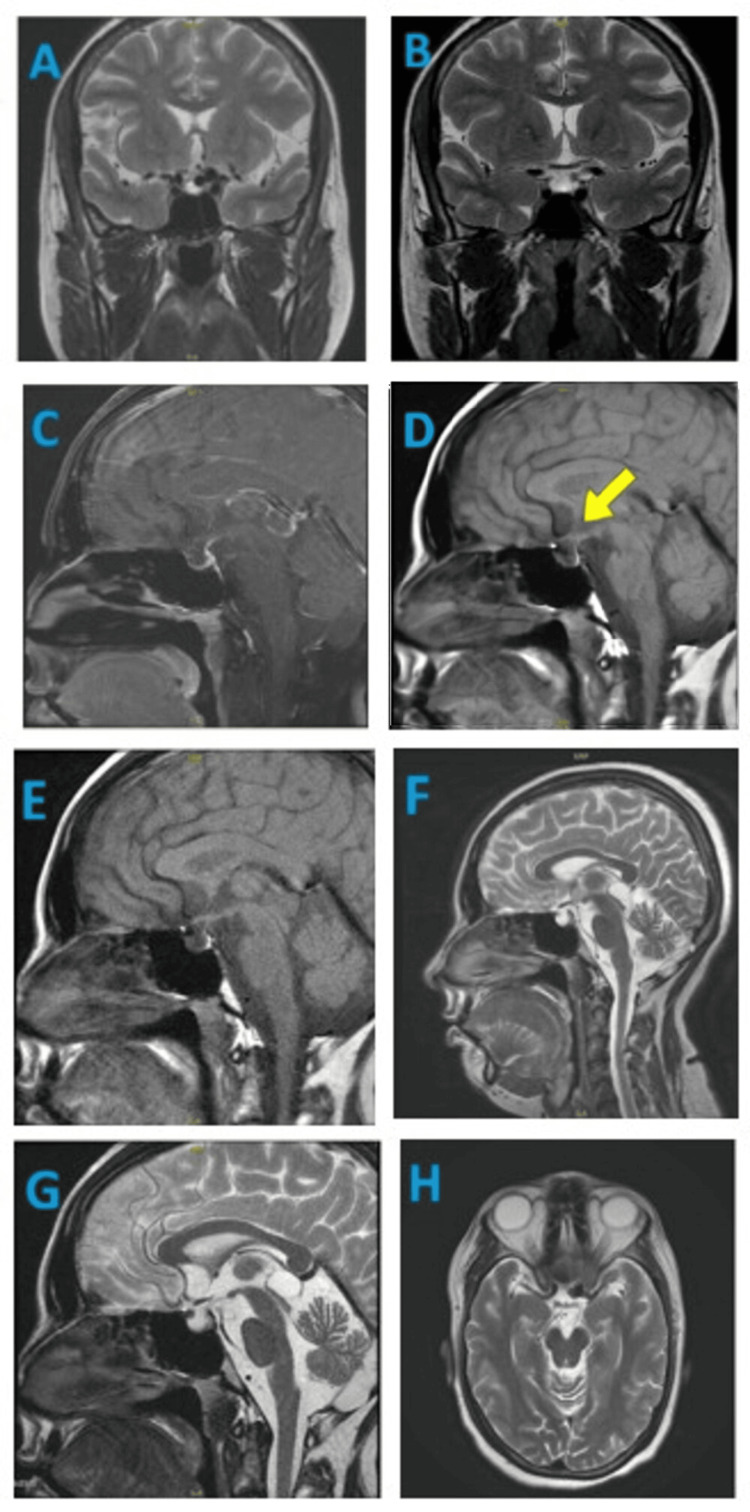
Magnetic resonance imaging of brain. Panels A–H: MRI of the brain demonstrating the sellar, suprasellar, and parasellar regions in multiple planes and sequences. Panels A and B: Coronal T2-weighted images delineate the pituitary gland, cavernous sinuses, optic chiasm, and adjacent temporal lobes. Panels C–E: Sagittal T1-weighted and contrast-enhanced images highlight the pituitary gland, infundibulum, optic chiasm, sphenoid sinus, and clivus. Panels F and G: Sagittal T2-weighted images demonstrate midline neuroanatomical structures, including the corpus callosum, third and fourth ventricles, brainstem, cerebellum, and pituitary gland. Panel H: Axial T2-weighted imaging provides visualization of the sellar and parasellar region, including the pituitary gland, cavernous sinuses, optic nerves, and temporal lobes.
